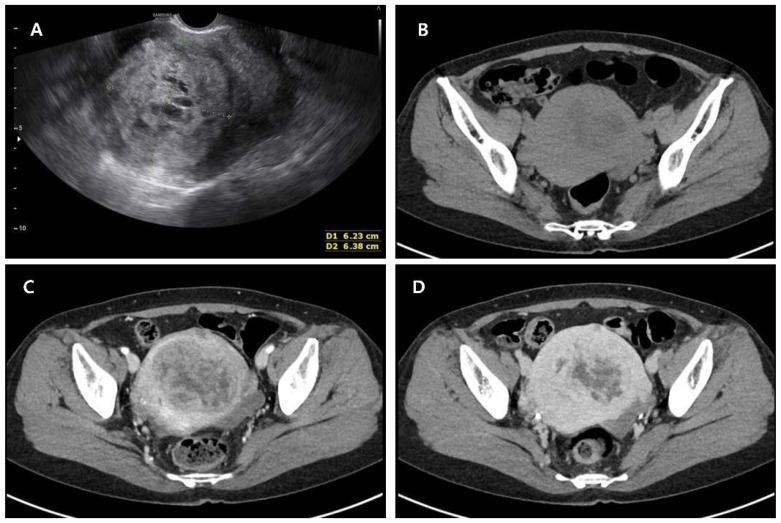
USG and Computed Tomography images of uterine mass. A 49-year-old postmenopausal woman (gravida 3, para 0) was referred to our institution for evaluation of a rapidly enlarging uterine mass that had been followed for four years as a presumed leiomyoma. She had experienced menopause three years prior and reported no abnormal vaginal bleeding or systemic symptoms. She had no significant underlying medical conditions and was not receiving any form of hormone replacement therapy. Transvaginal ultrasonography revealed an enlarged, heterogeneous echogenicity with irregular internal architecture, including focal cystic changes within the uterus, measuring approximately 6.23 × 6.38 cm in maximal diameter (A). On axial pre-enhanced CT image, a well-defined uterine mass with heterogeneous iso- to hypodense attenuation was seen, showing central low-density areas (B). On contrast-enhanced axial CT, the lesion showed heterogeneous enhancement, characterized by alternating solid enhancing areas and non-enhancing hypodense regions (C). On a delayed contrast-enhanced axial CT, the mass demonstrated a persistent enhancement of the solid areas of the tumor, with non-enhancing central portions corresponding to degeneration or necrosis (D). Ultrasonography revealed a large, well-circumscribed, heterogeneously hypoechoic uterine mass with cystic degeneration. CT imaging demonstrated a solid lesion with heterogeneous enhancement and central hypodense areas, and findings were compatible with a degenerative leiomyoma. However, these imaging features are not specific and may also be seen in uterine sarcomas, limiting the diagnostic value of ultrasound and CT in differentiating benign from malignant smooth muscle tumors.
